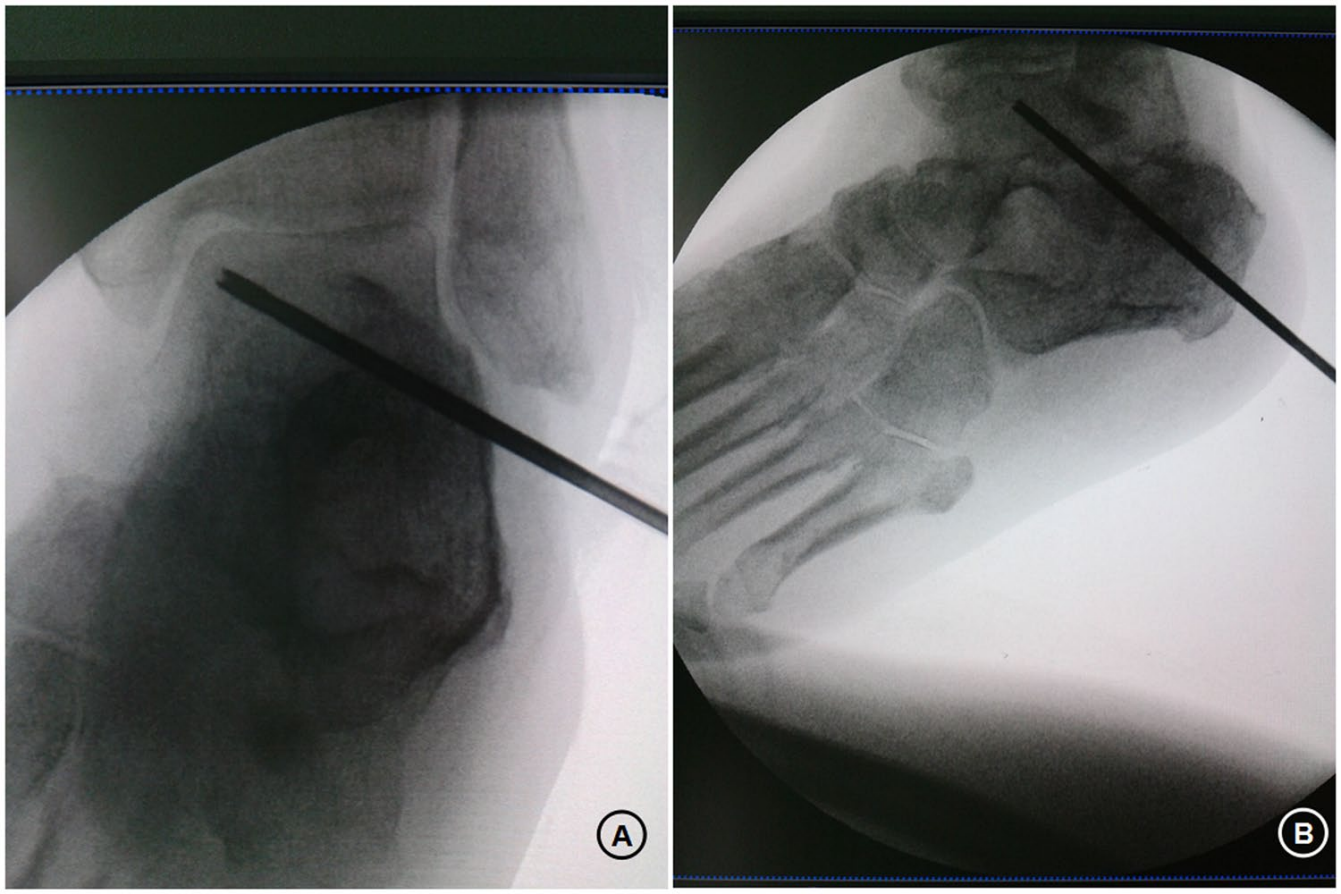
Fluoroscopy-guided positioning of the pilot Kirschner wire into the center of a medial osteochondral lesion (OCL) in AP and lateral plain view radiographs. Guided by the Kirschner wire, the hollow trephine was gently passed through the sclerotic zone of the osteochondral lesion (OCL), controlled by fluoroscopy in AP (A) and lateral (B) plain view radiographs. Debridement of the osteochondral lesion (OCL) through the drilling hole. The aspirate from the ilium could be injected under the guidance of the hollow trephine to the OCL site. Note: The patient consented to publish the specific information.
